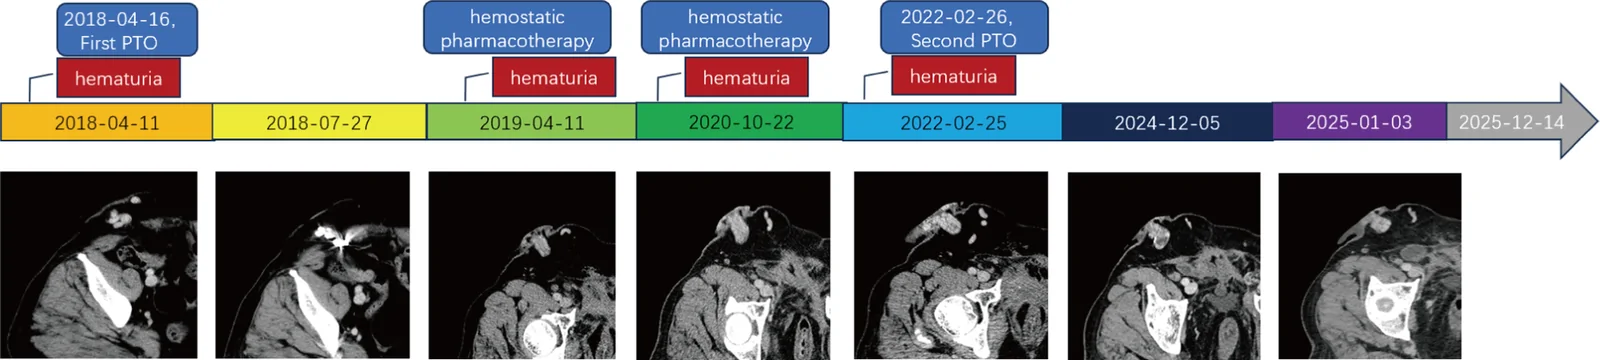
Long-term follow-up: contrast-enhanced CT obtained at the 7-year follow-up showing minimal, nonprogressive residual peristomal varices without active bleeding. The timeline above the images indicates the key clinical events: first PTO (April 2018), recurrences (16 and 20 months), second PTO (February 2022), and the 7-year follow-up CT (December 2025). CT = computed tomography, PTO = percutaneous transhepatic obliteration.
